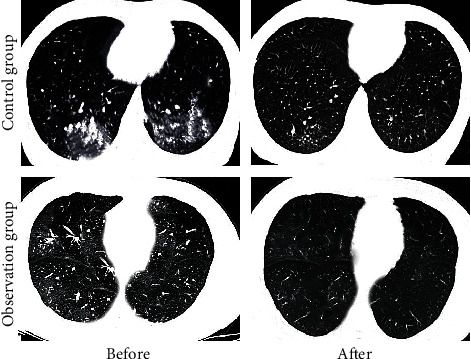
HRCT images before and after treatment.
